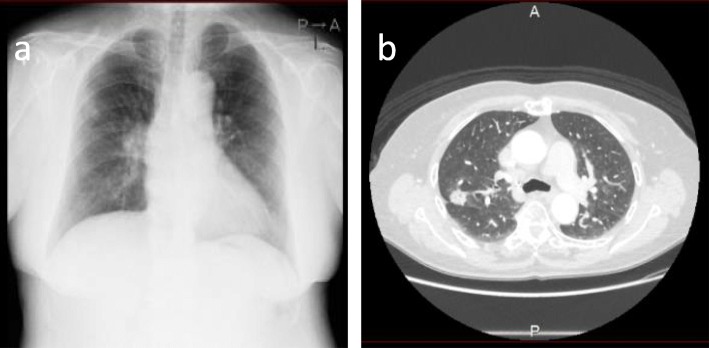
An abnormal chest shadow can be seen on a chest roentgenogram in the right upper lung field (a). A chest computed tomography scan showed an abnormal lesion, 2.6 × 2.4 cm in size, in the right upper lobe (b)
